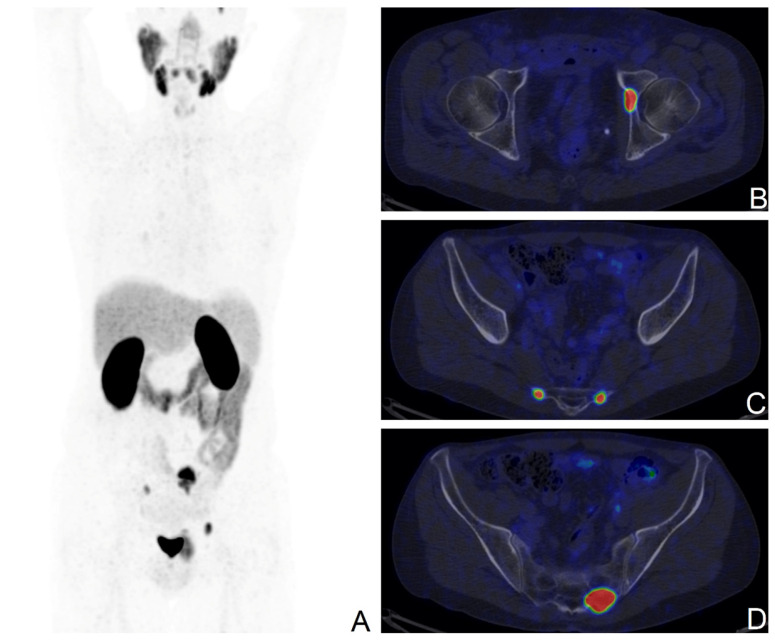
[^68^Ga]Ga-PSMA-11 PET/CT scan of a BCR patient with the ISUP score of 5, pT3a after RP; PSA levels at the time of the scan: 1.43 ng/mL; Maximum Intensity Projection image (A), fused axial PET/CT views showing secondary bone lesions at the left acetabulum (B), and at the sacrum bilaterally (C,D).
